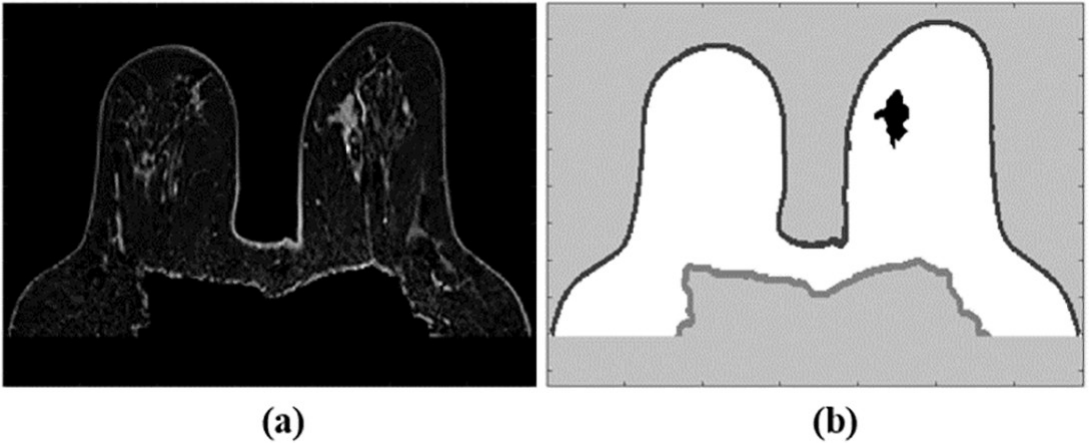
Example of a MRI exam and corresponding label map (Exam 11 of the repository). (a) Axial view of a T1-w_Dixon-W_pre-processed image. (b) Axial view of the corresponding Label_map_simple.
